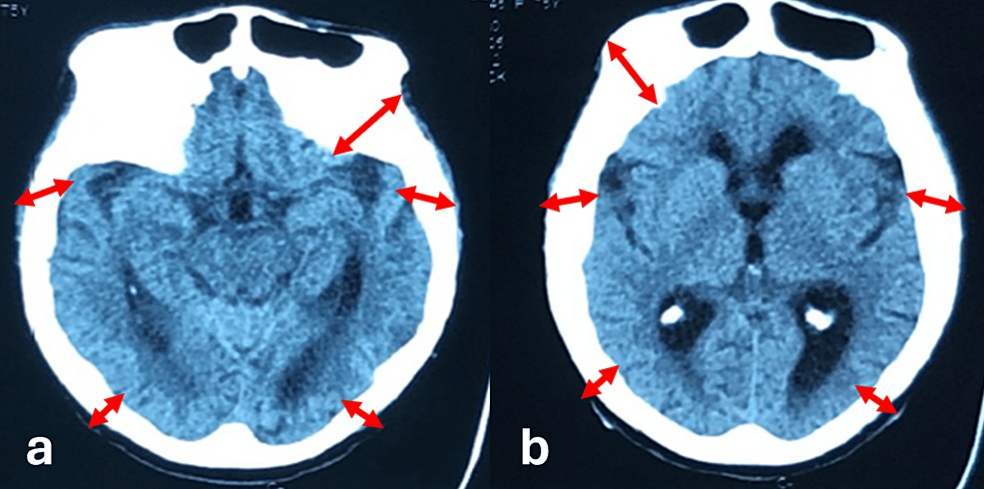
Brain axial CT scan without contrast Generalized thickening of the skull bones (red arrows).
